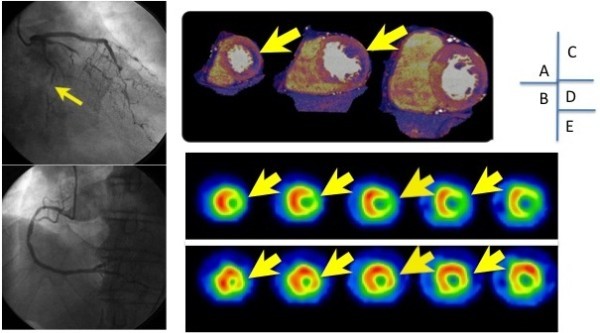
Case (CAD = coronary artery disease, SPECT = single photon emission computed tomography). Case was a patient with asymptomatic CAD. Severe stenosis is seen in the left circumflex (panel A), and RCA is normal (panel B). Stress dual-energy imaging shows ischemia in the lateral wall (panel C), which correlated with the lateral wall ischemia seen on SPECT (panels D and E).
